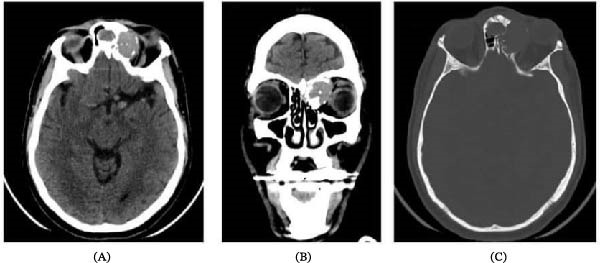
Nonenhanced brain CT images: (A) axial soft tissue window, (B) coronal soft tissue window, and (C) axial bone window; showing a well‐defined slightly hyperdense expansile lesion epicentered at the left anterior ethmoid air cells and left frontal sinus with extension into the medial extraconal space abutting the left medial rectus muscle. Several foci of calcification are seen within the lesion. The expansion of the lesion at the bone window (C) with marked thinning and destruction of the left medial orbital wall. Features are of aggressive lesions.
